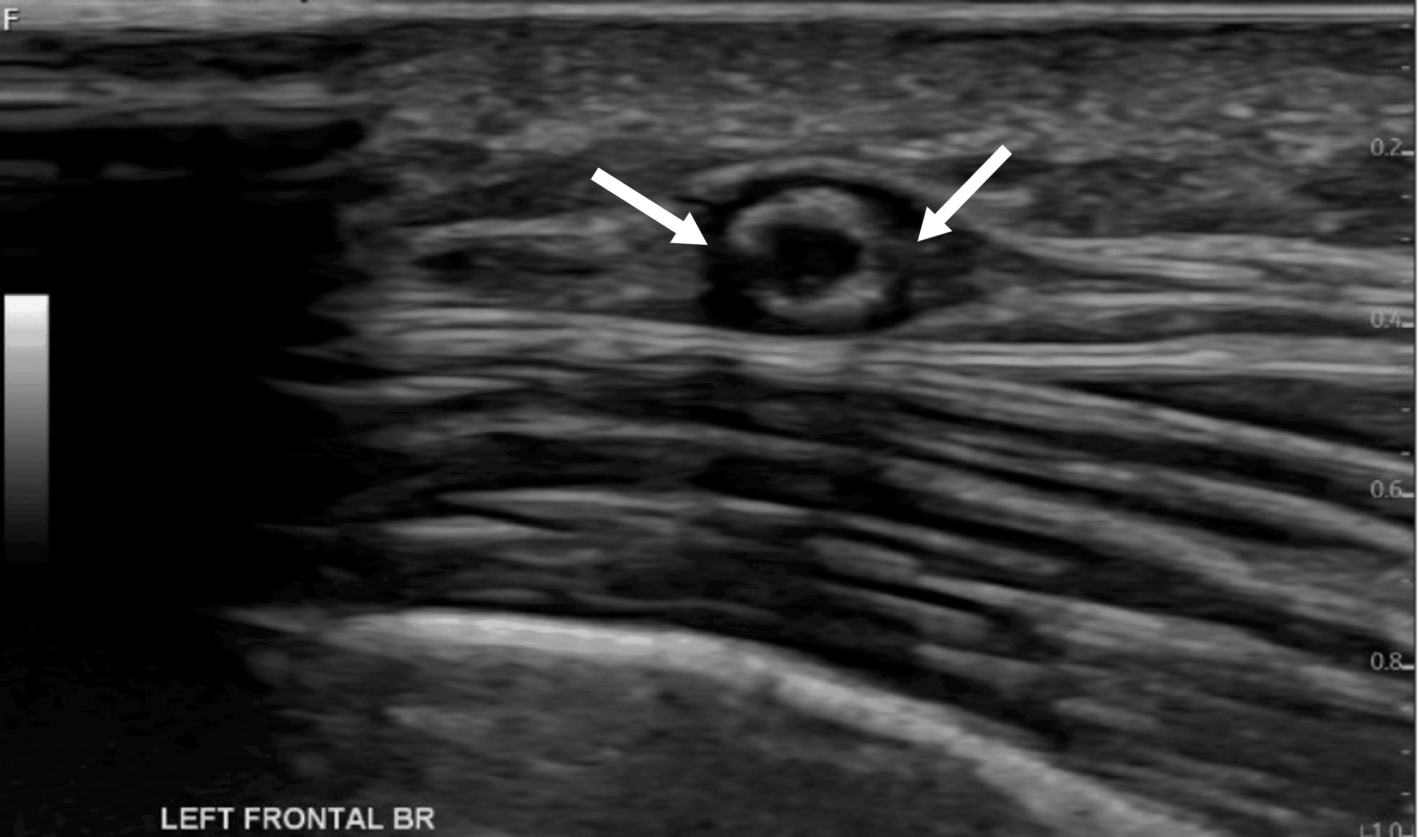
The left parietal branch US Doppler There is sonographic evidence of a dark periluminal halo sign (arrows), highly suggestive of giant cell arteritis US: ultrasound
